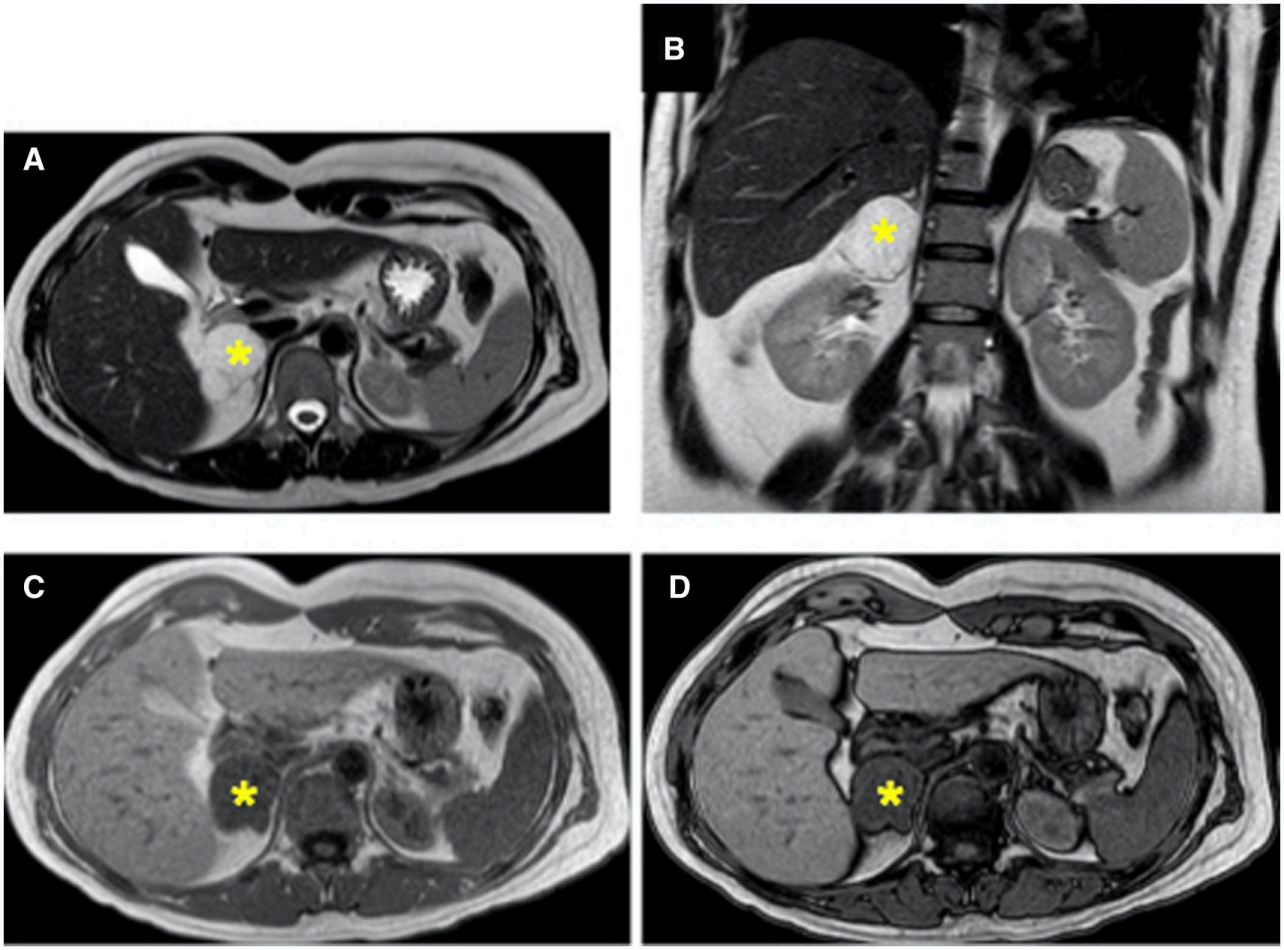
Abdominal MRI shows a right adrenal mass (yellow asterisk) with high signal intensity on T2-weighted axial (A) and coronal (B) images, and an absence of signal intensity drop out on out-of-phase compared with in-phase images (C and D).
